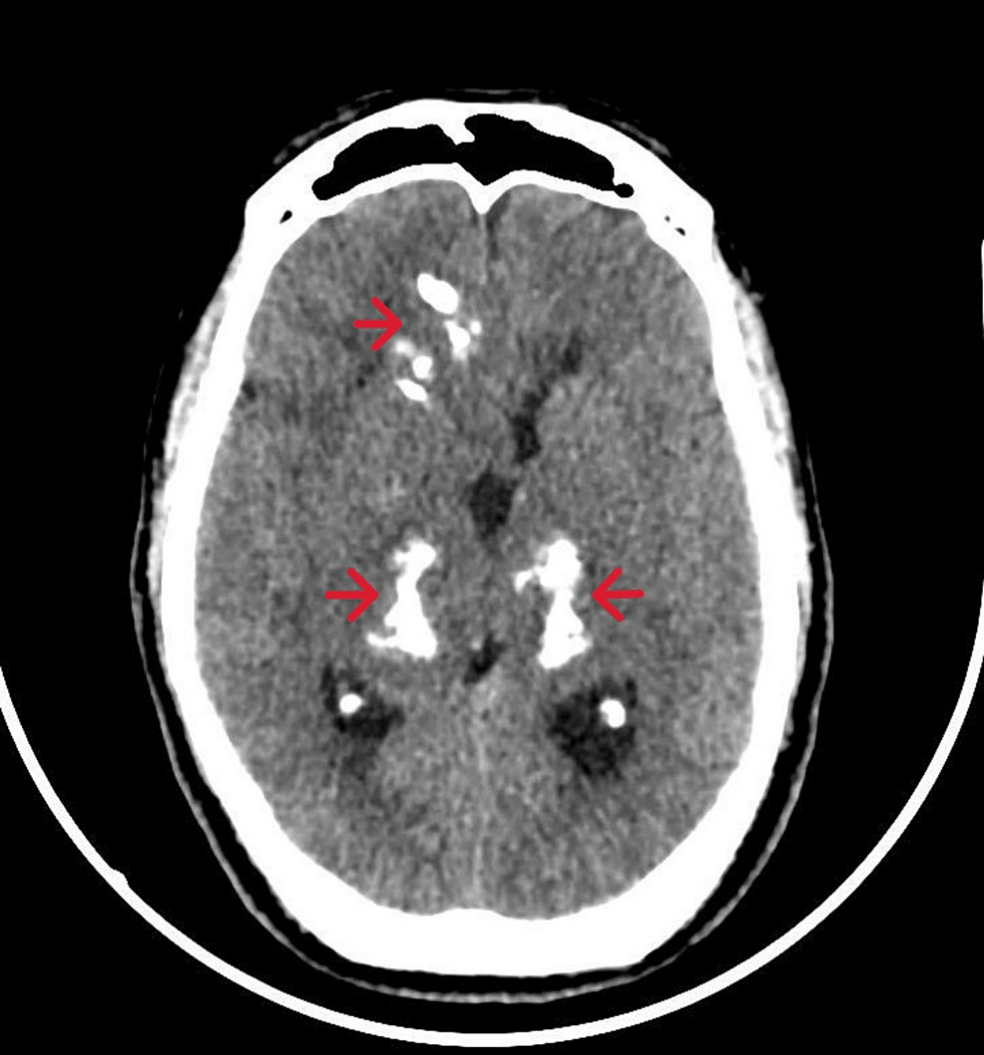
Brain CT - Diffuse areas of calcifications
